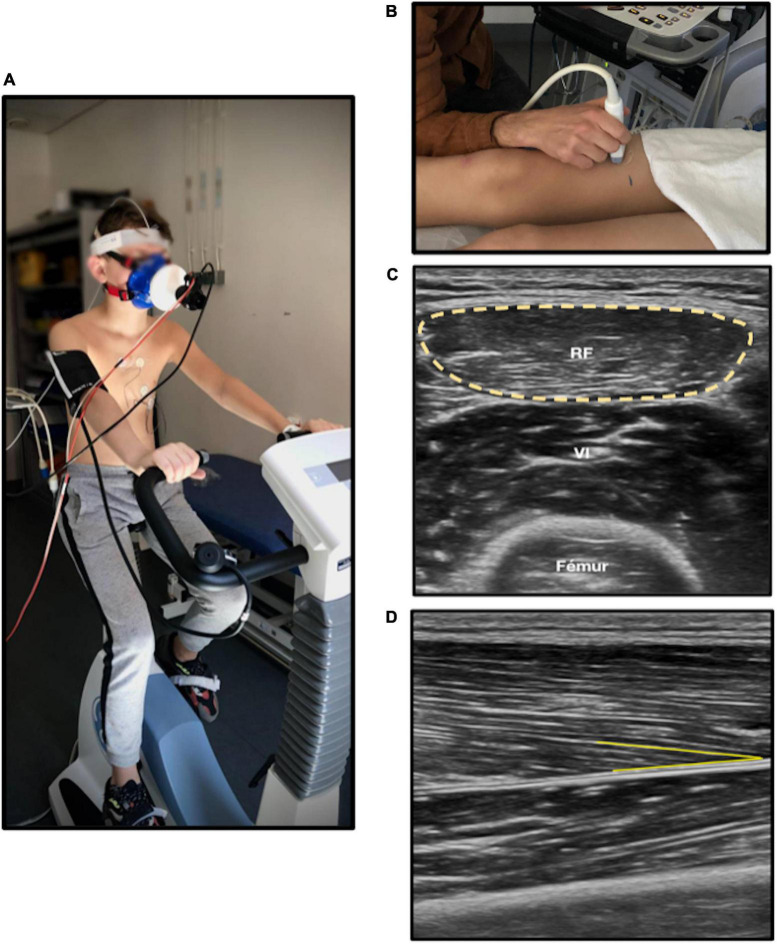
Cardiorespiratory and muscle fitness analysis. (A) Cardiopulmonary exercise test on ergocycle. (B) Muscular ultrasound on right leg. (C) Anatomical cross-sectional area (yellow circle) of rectus femoris measured with ultrasound. (D) Pennation angle (yellow line) of right vastus lateralis; RF, Rectus femoris. The chosen anatomic site was at two third of the length from iliac spine anterior superior to upper edge of the patella on right leg. The probe was put in transversal plane and longitudinal plane for cross-sectional area and pennation angle, respectively.
